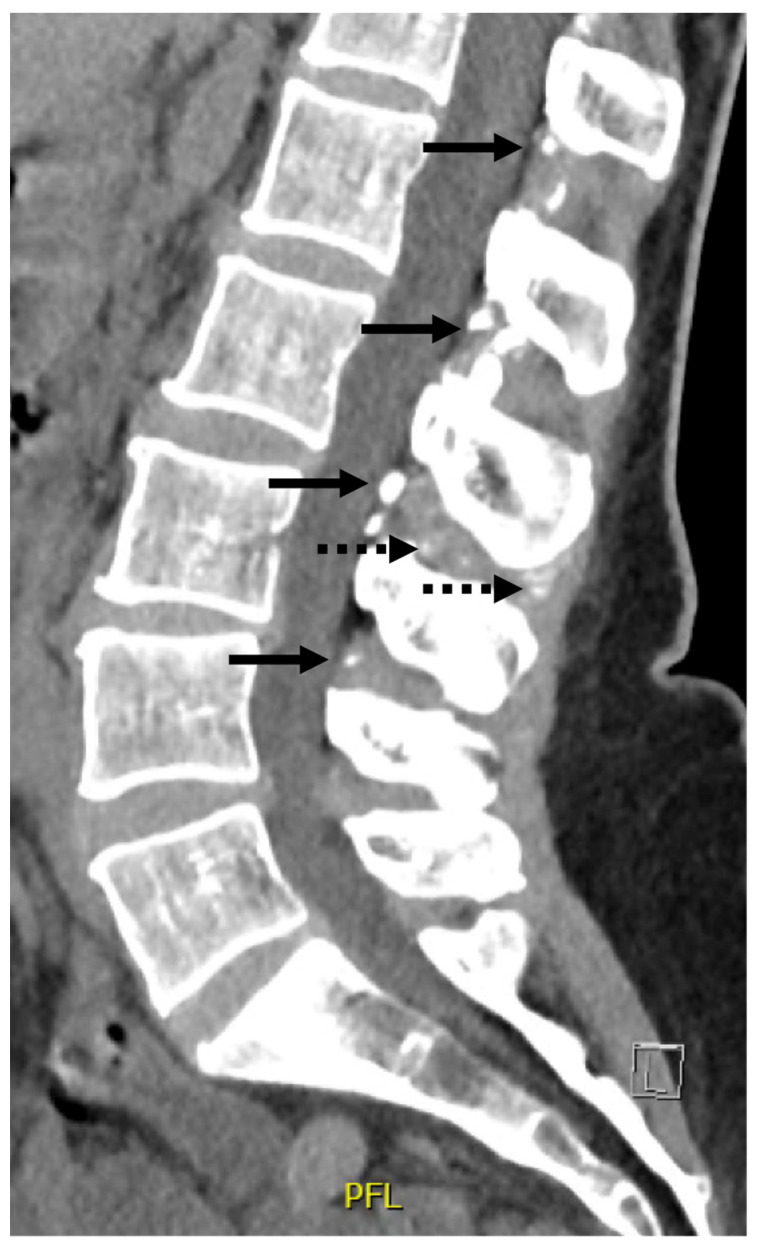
Lumbar CT scan. The lumbar CT scan, performed three months before the consultation, demonstrated diffuse degenerative disc disease with atypical heterotopic calcifications of the yellow ligament (arrows) and interspinous ligaments (dotted arrows).
